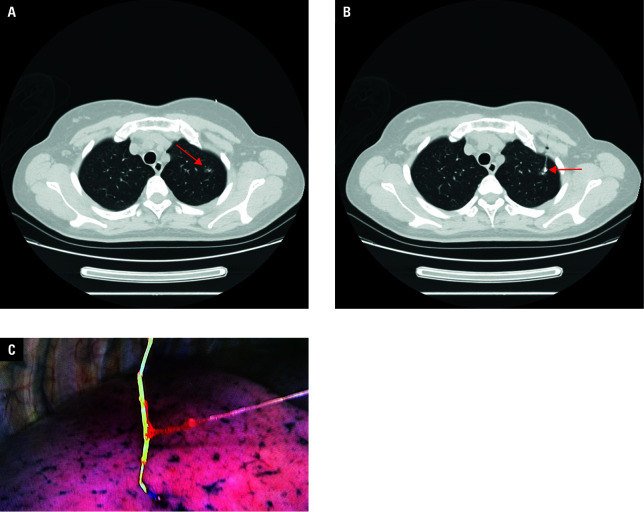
Periprocedural imaging of a patient undergoing computed tomography–guided soft hook-wire (HW) localization; A – a ground -glass nodule (GGN) located at the left upper lobe (arrow); B – placement of the soft HW near the GGN (arrow); C – soft HW visualization during video-assisted thoracoscopic surgery
